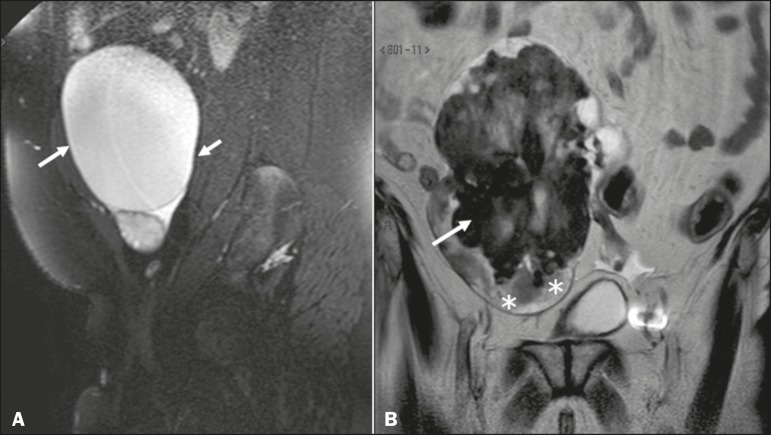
Sagittal and coronal T2-weighted MRI sequences (A and B, respectively). A: Preserved ovarian volume and an adjacent hyperintense expansile cystic formation consistent with a paraovarian cyst (arrows). B: Image of another patient with an enlarged ovary due to expansile, hypointense, heterogeneous fibroma-like formation (arrow). Note also the edema at the periphery of the ovarian parenchyma (asterisks).
